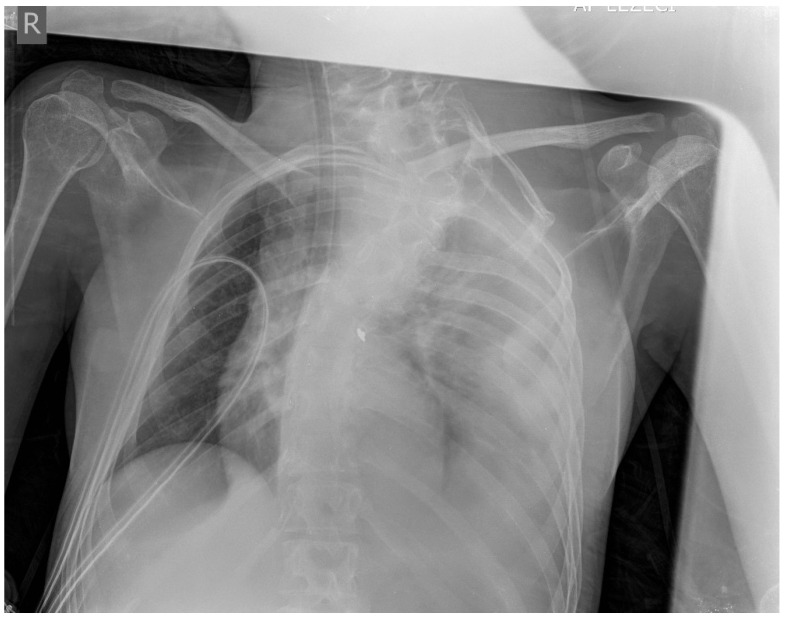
Frontal chest radiography demonstrates complete re-expansion of the lungs.
